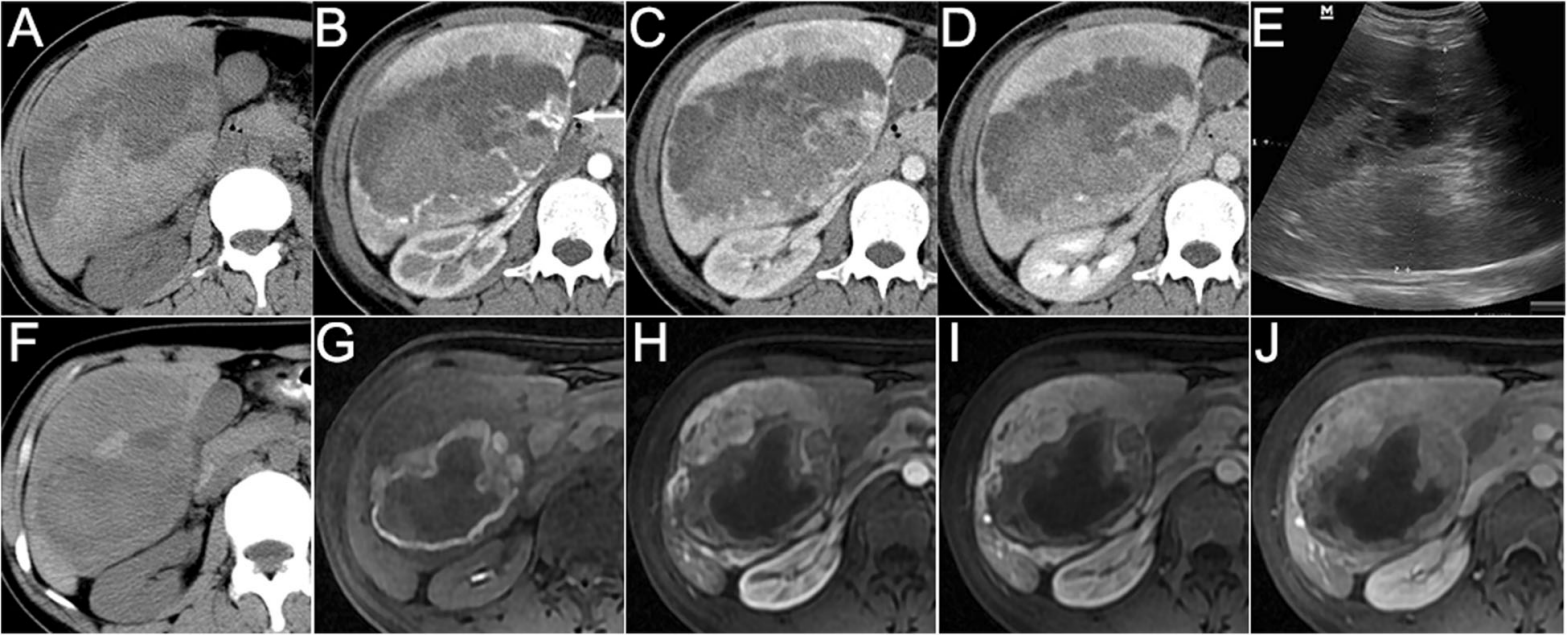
A 29-year woman with clear-cell myomelanocytic tumor of falciform ligament/ligamentum teres. This patient presented acute pain of upper abdomen and was hospitalized in the emergency department. The non-enhanced CT (a) showed a large heterogeneous mass in the right hepatic lobe with high and low attenuation. Contrast-enhanced CT images (b, arterial phase; c, portal venous phase; d, delayed phase) demonstrated heterogeneous enhancement with necrosis and hemorrhage within the mass. Note the mass was surrounded by dysmorphic vessels (arrow) on arterial phase image. Ultrasound (e) showed an ill-defined, large hyperechoic mass with hypoechoic region within the lesion in the right lobe of liver. The patient was performed percutaneous artery embolization. One month later, she underwent CT and contrast-enhanced MRI to reevaluate the mass. Necrosis, hemorrhage and heterogeneous enhancement were also identified on non-enhanced CT (f) and contrast-enhanced MRI (g, mask T1WI; h, arterial phase; i, portal venous phase; j, delayed phase) images. Note the tumor diameter was decreased from 130 mm to 97 mm
